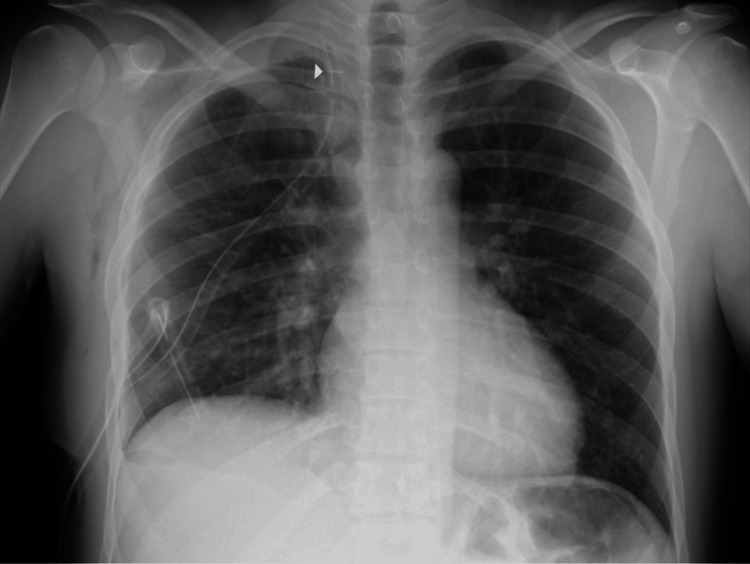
Postoperative chest radiograph Postoperative chest radiograph showing satisfactory re-expansion of the right lung with a small residual pleural effusion. The white arrowhead indicates the surgical clip applied to the disrupted apical adhesion during surgery.
